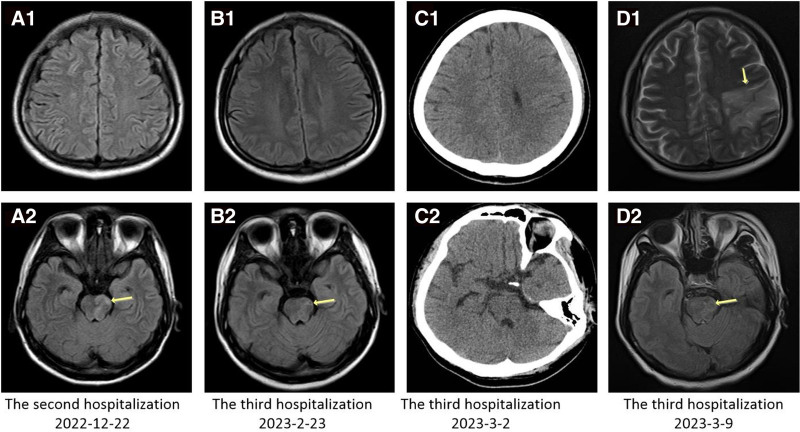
Imaging changes in the patient’s head. (A) During the second hospitalization, a brain MRI T2-Flair revealed multiple high signals in the brain stem. (B) Brain MRI T2-Flair at the beginning of the third hospitalization showed multiple high signals in the brain stem, which were slightly improved compared to those observed during the second hospitalization (Panel A). (C) The patient’s condition suddenly deteriorated during the third hospitalization, and a brain CT scan showed no apparent abnormalities. (D) Subsequent brain MRI T2-Flair during the third hospitalization showed a large area of acute cerebral infarction in the left frontal and parietal lobes, as well as multiple high signals in the brain stem. CT = computed tomography, MRI = magnetic resonance imaging.
